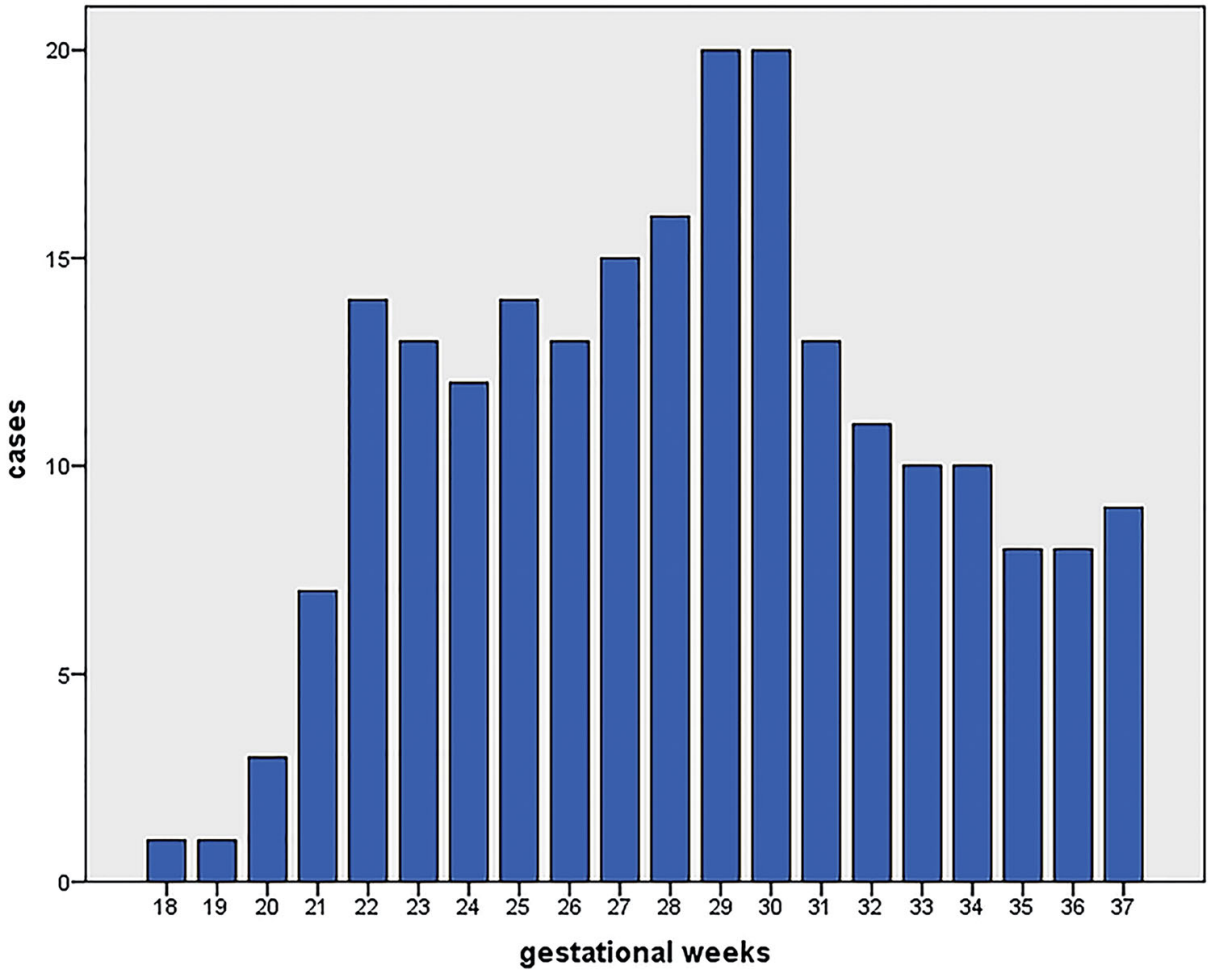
Distribution of 218 subjects included in our study by gestational age at the time of prenatal MRI examination.
